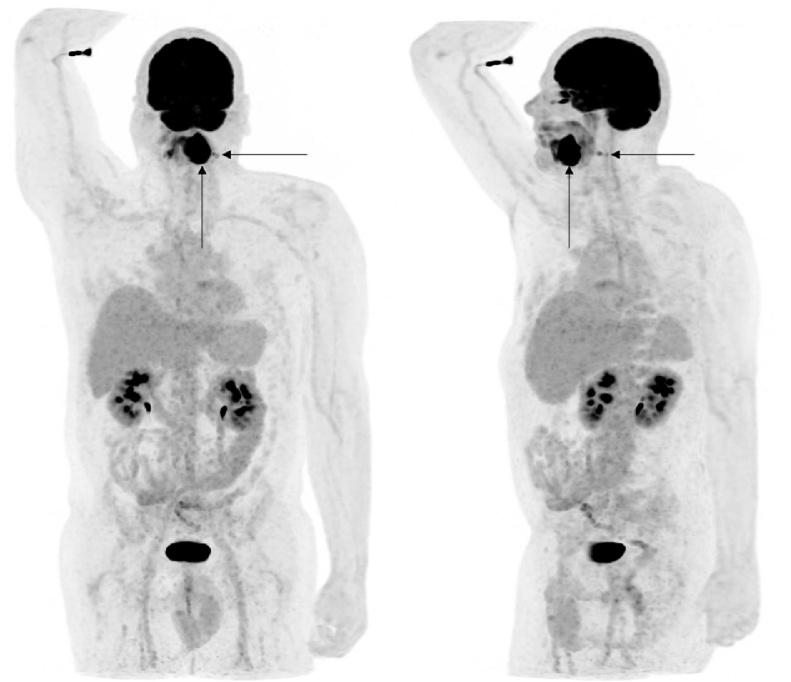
Two different MIP (Maximum intensity projections) of the FDG-PET-data after CTAC (CT-based attenuation correction), showing the local finding in the left mandible and the two lymph node metastases ipsilateral, no distant metastases.
